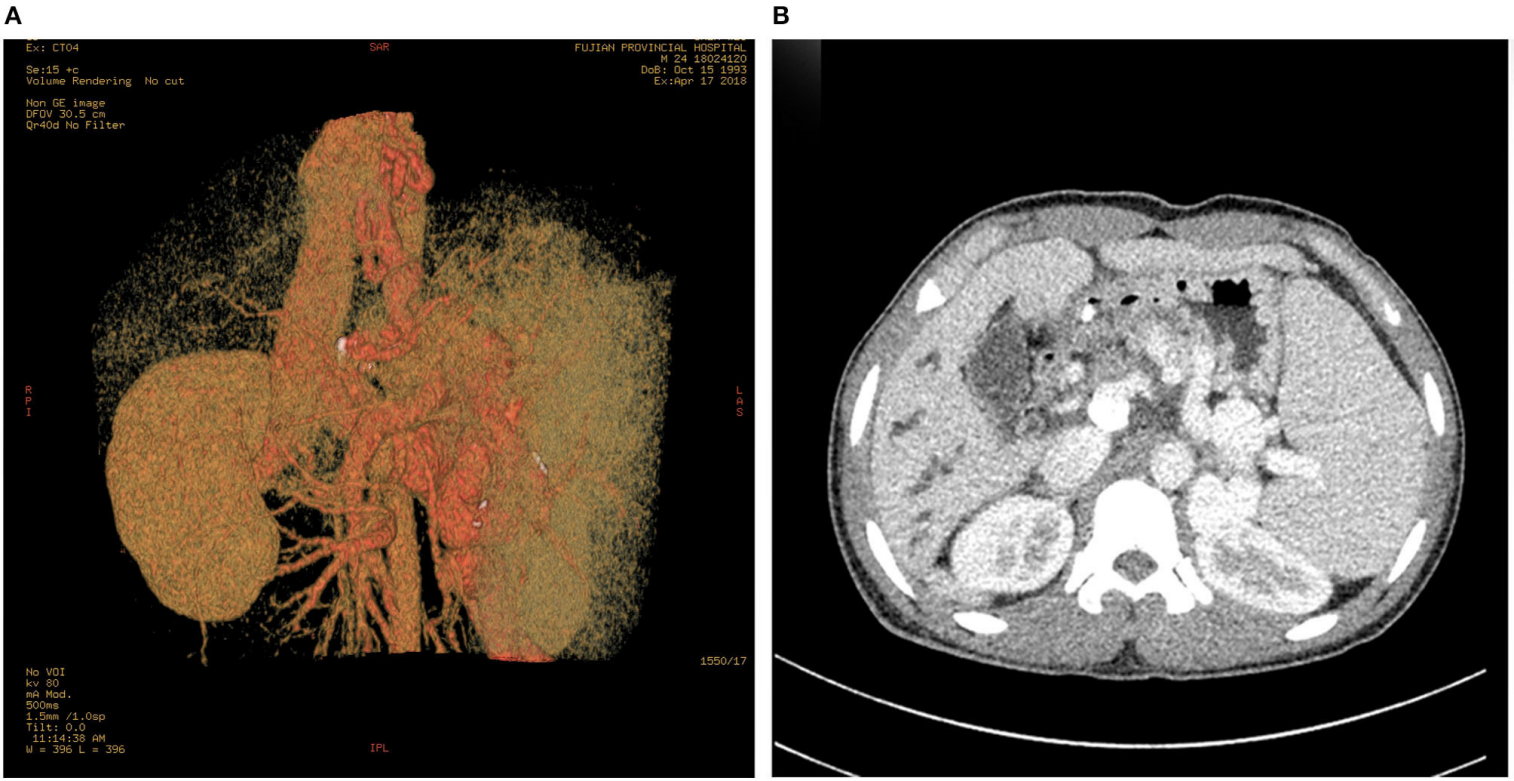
PV-CTA shows that one branch of SV flows into IVC *via* LRV (A). The other branch of SV merges with SMV into PV, the intrahepatic branch of PV is slim, and the proximal trunk of PV is short and calcified (B). The esophageal and gastric fundus vein (A), and the SV are dilated and tortuous (B). Dilatation of intrahepatic bile duct (B). PV-CTA, portal vein-computed tomographic angiography; SV, splenic vein; IVC, Inferior vena cava; LRV, left renal vein; SMV, superior mesenteric vein.
